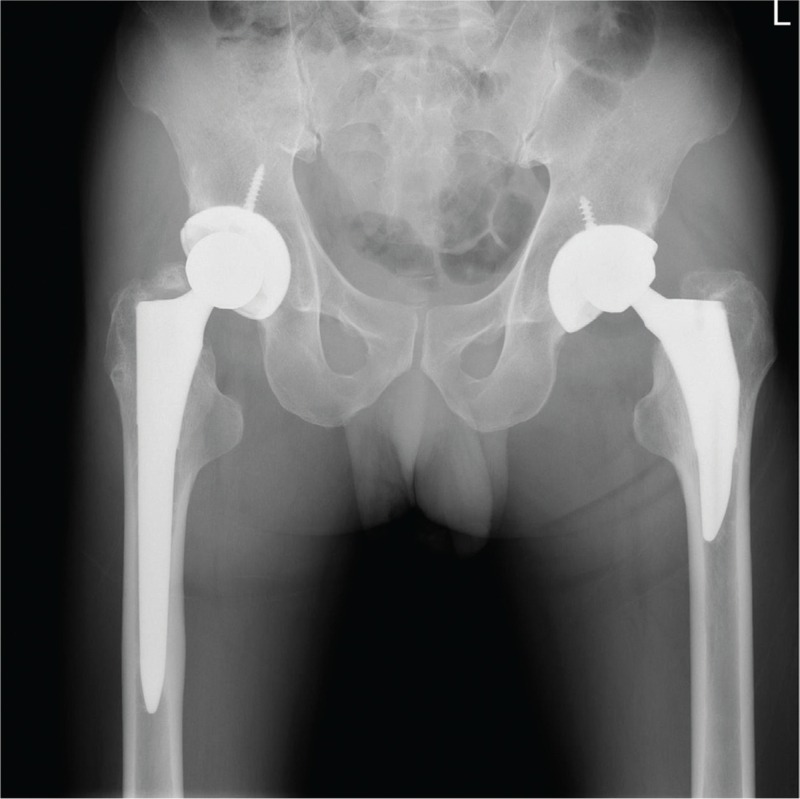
Plain AP radiograph taken 1.5 years post-surgery showing eccentric elevation of prosthetic femoral head. AP = anteroposterior.
